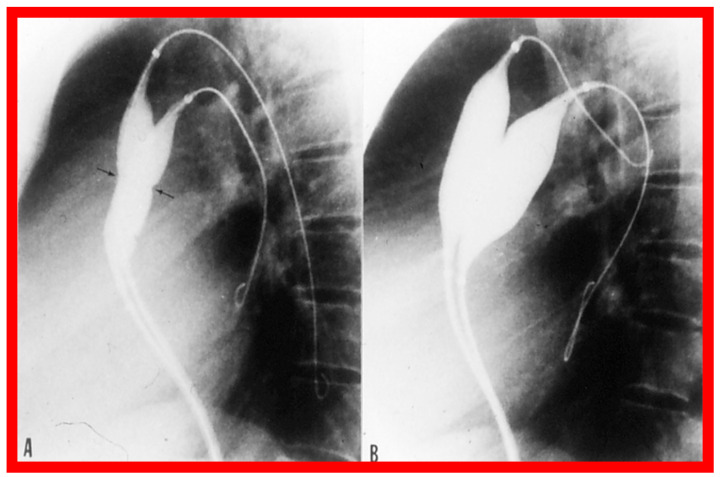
Selected cine-radiographic frames in lateral view, demonstrating two balloon catheters placed across the pulmonary valve, showing “waisting” of the balloons (arrows) during the initial phases of balloon inflation (A), which was completely abolished after the complete inflation of the balloons (B). Reproduced from Reference [62].
